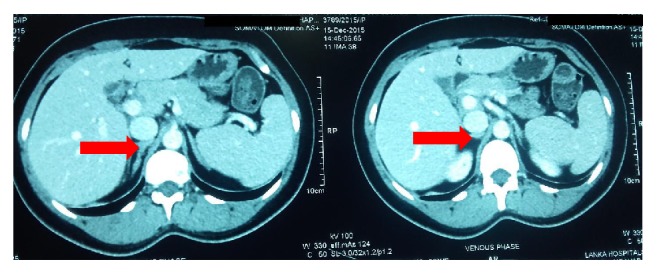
Contrast enhanced CT abdomen showing right-sided adrenal adenoma (red arrow).
